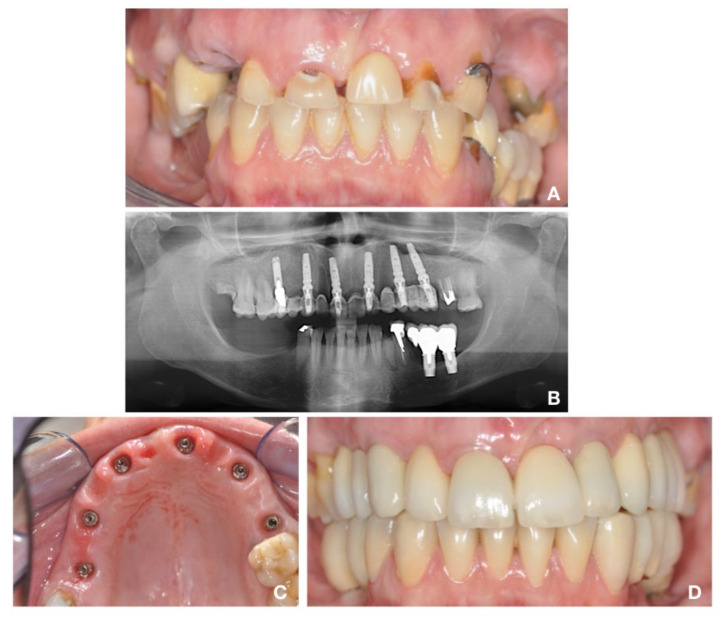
Full arch restoration in fresh extraction sockets, immediately loaded with temporary abutment and prosthesis. (A) Clinical situation before teeth extraction. (B) Radiographic and (C) clinical evaluation after 10 days from implants insertion: soft tissues around the implants did not show signs of inflammation. (D) Clinical evaluation after 5 years follow-up: interdental papillae appeared well preserved and no soft tissues retraction occurred.
